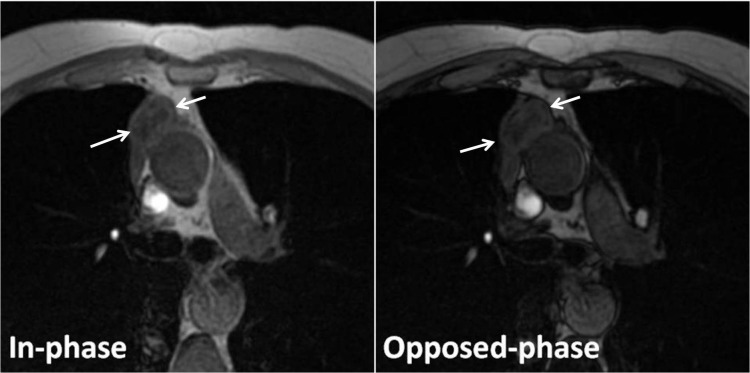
Thymoma in a 52-year-old man with myasthenia gravis. Transverse in-phase (155/4.6) and opposed-phase (155/2.1) gradient-echo T1-weighted MR images demonstrate no change in signal intensity of the lesion on the opposed-phase image relative to the in-phase image (arrows). The CSR is of 1.050.
